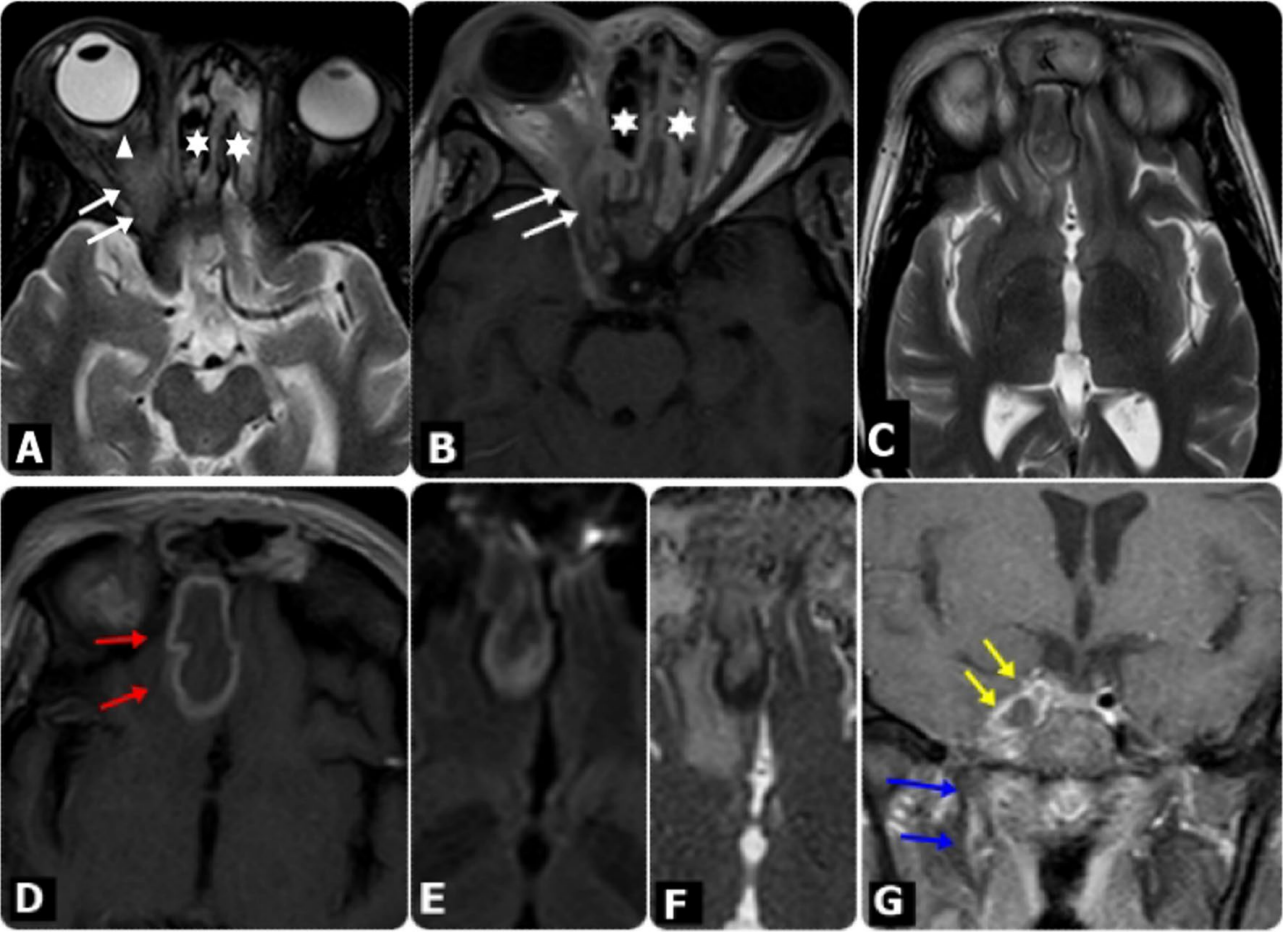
MRI findings in female patient, 36 years old, with chronic renal disease presented mainly with headache and altered mentation, in addition to Rt. eye proptosis, with periocular and eyelids edema, following COVID-19. A Axial T2 SPIR, B Axial post-contrast T1W images showed ethmoidal sinusitis (Asterisks), with extension of the inflammatory process to the Rt. orbit resulting in proptosis of Rt. eye globe, with posterior globe tenting (white arrow head), as well as Rt. periorbital and eyelids edema. There is retro-orbital fat stranding and heterogeneously enhancing soft tissue at the orbital apex (white arrows), that extend to the Rt. cavernous sinus. C Axial T2 WI showed extension of the inflammatory process to the Rt. basi-frontal lobe, exhibiting an area of abnormal signal intensities, that shows peripheral ring enhancement (red arrows) on axial post-contrast T1WI (D), presenting restricted diffusion on DW images at b-value 1000 (E) and apparent diffusion coefficient (ADC) map (F) in keeping with cerebral abscess. G Coronal post-contrast fat-saturated T1WI showing mild enlarged right cavernous sinus with patchy heterogeneous enhancement, with attenuated cavernous portion of the Rt. ICA (yellow arrows), associated with inflammatory spread to the Rt. infra-temporal fossa region (blue arrows)
